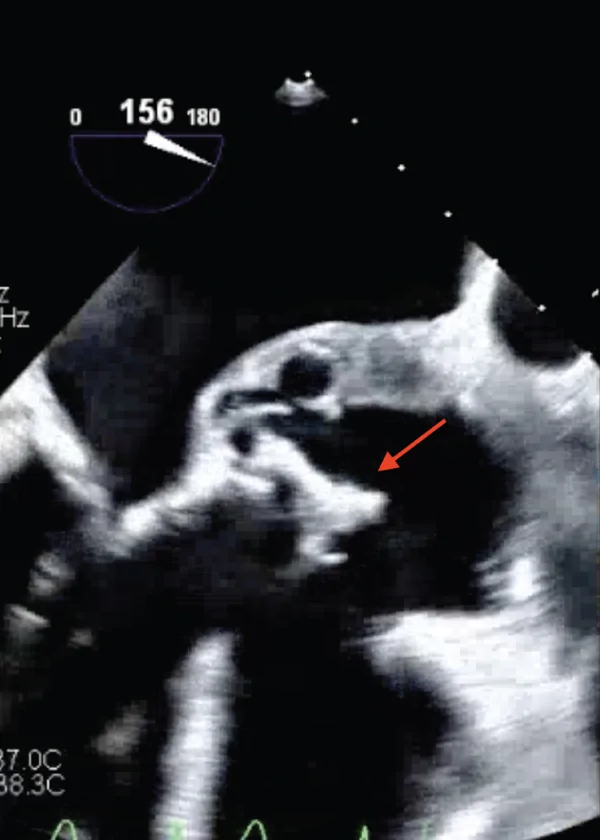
Transesophageal echocardiography demonstrating a large, multilobulated, mobile echogenic mass attached to the bioprosthetic aortic valve, consistent with vegetation.
